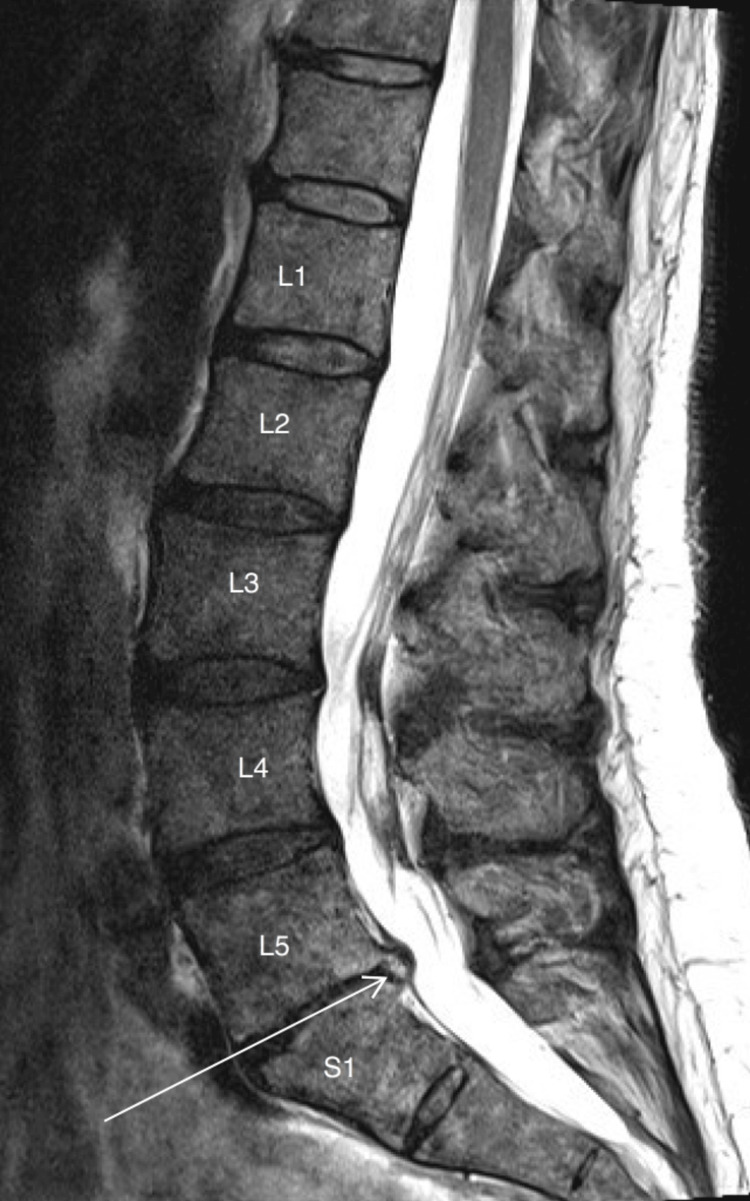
Lumbar MRI showing the osteophyte complex (arrow) at the level L5-S1 MRI: magnetic resonance imaging
